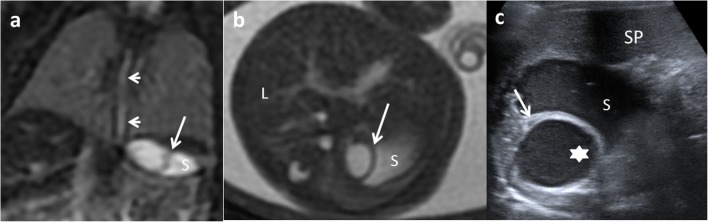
A 22-week-old fetus with an abdominal cyst seen on ultrasound is studied with MR. a Coronal fetal FIESTA MR: a cystic lesion is seen next to the stomach (*S*). Oesophageal lumen is seen (*arrows*). b FIESTA transversal MR: the hypointense wall of the suspected gastric duplication cyst (*arrow*). *L* liver, *S* stomach. c Postnatal abdominal US view: the lesion (*star*) imprinting the gastric wall (*arrow*). The content of the stomach is seen (*S*). *SP* spleen. Surgical findings: duplication cyst of the oesophageal-gastric transition
